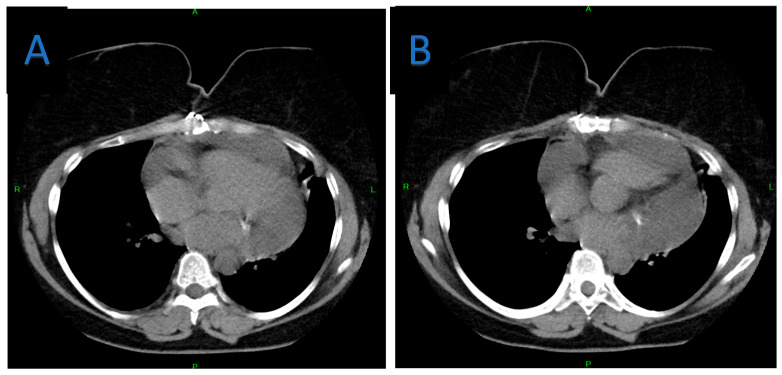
Axial chest CT images at one-year follow-up. (A,B) show evidence of pericardial mass, effusion.
